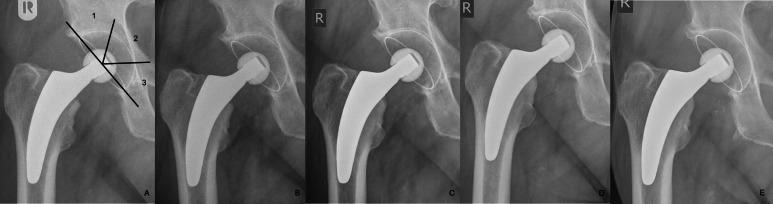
Radiographs of 53-year-old male patient with signs of a lucent line. a) Immediately postoperation with DeLee and Charnley zones, b) six weeks postoperatively, c) follow-up at one year, d) five years, and e) ten years.
